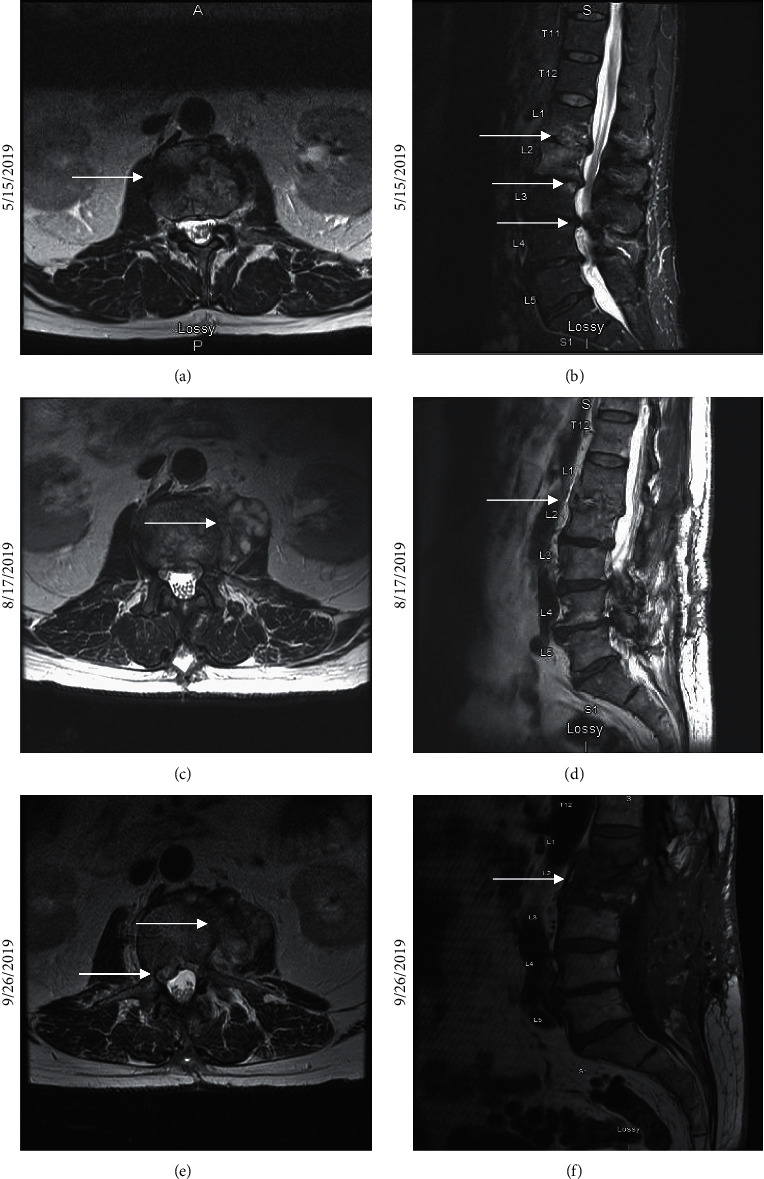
(a) An axial T2-weighted MRI image of morphological changes at the L1-L2 level presumed to be from degenerative process but difficult to distinguish from early infection. (b) A sagittal STIR MRI image showing multilevel degenerative changes resulting in severe canal stenosis particularly at the L2-L3 and L3-L4 levels. The morphological changes observed in Figure 1(a) are also visible. (c) An axial T2-weighted MRI image showing a heterogenous left paraspinal spinal mass with solid and cystic components. These findings raise concern for a potentially neoplastic process superimposed on an atypical infection. (d) A sagittal T1-FLAIR MRI image showing progressive, extensive degeneration at the L1-L2 and L3-L4 levels since prior study, suggesting infectious etiology. (e) An axial T1 FS post-MRI image showing 2 new small nonenhancing abscesses within the left psoas muscle and right L2-L3 paraspinal phlegmon. This epidural phlegmon, together with the microabscesses, caused an apparent impingement of the right L2 nerve at the right proximal margin of the right L2-L3 neural foramen. (f) A sagittal T1 MRI image showing L1-L2 discitis and vertebral, endplate osteomyelitis of L2.
